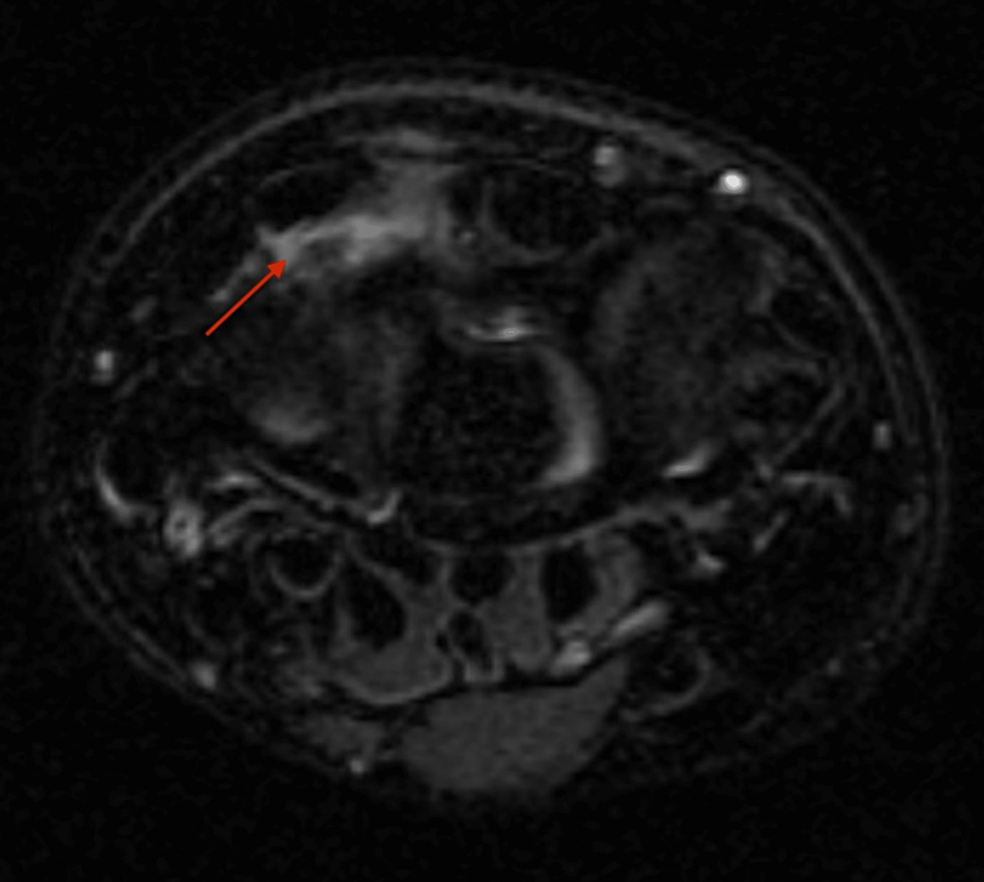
Axial T2-weighted images of the patient’s right wrist Red arrow: Peritendinous edema (external to the tendon sheath) surrounding 2nd and 3rd extensor compartments.
